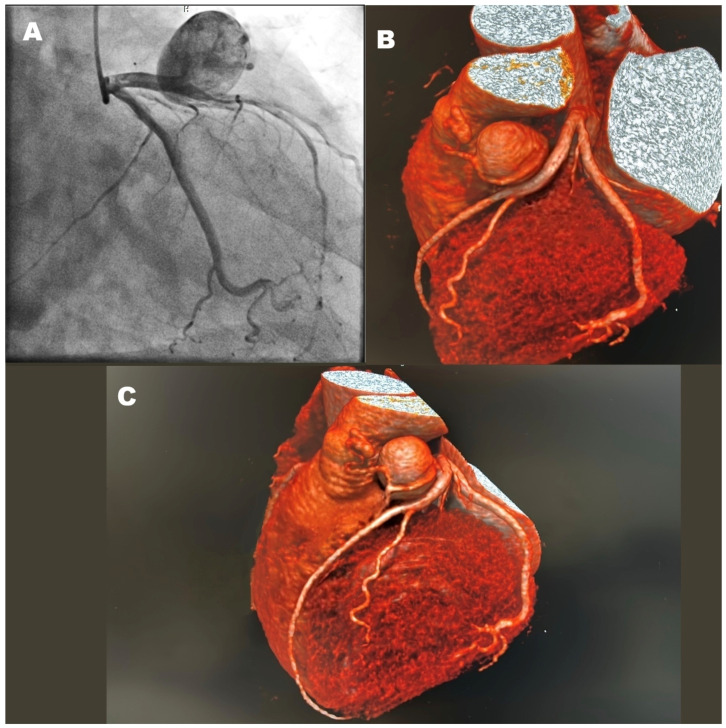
Giant aneurysmatic fistula between the left anterior descending coronary artery and the pulmonary artery (LAD), demonstrated by coronary angiography (A) and three-dimensional computed tomography reconstruction viewed from different angles (B,C).
